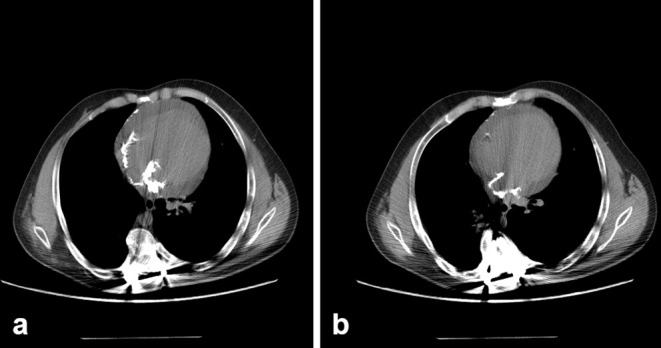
Non-enhanced CT, showing extensive pericardial and atrial calcifications.
